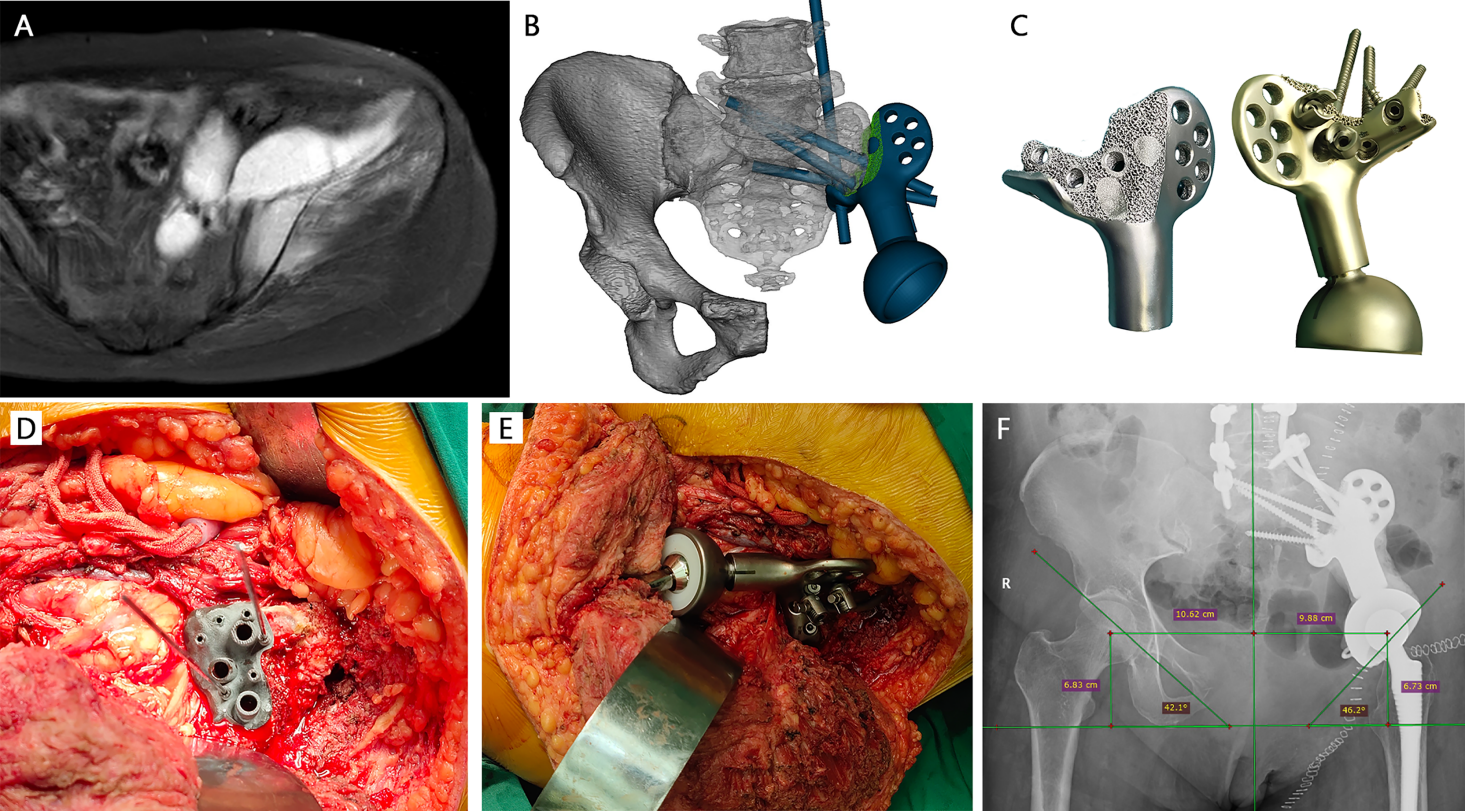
A 53-year-old female patient with chondrosarcoma. (A) Preoperative MRI showed the invasion of the sacroiliac joint. (B) The design of Type A prosthesis (green part: porous structure; blue part: solid structure). (C) The 3D-printed prosthesis with lattice bone contact surface. (D) Intraoperative installation of patient-specific drill guide at the auricular surface. (E) Installation of 3D-printed prosthesis. (F) Immediate x-ray after surgery showed that the prosthesis was fitted precisely.
